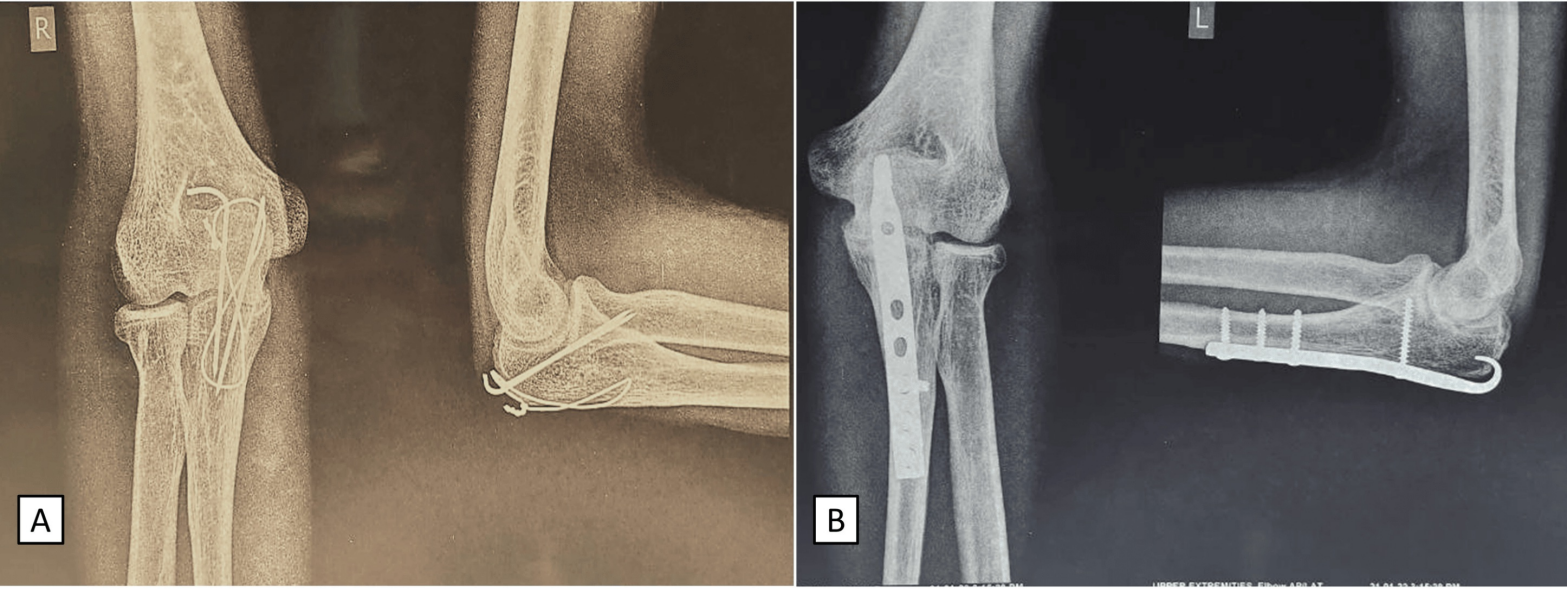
Postoperative radiographs of the left and right elbows taken six months after surgery (A) Radiograph showing the union of the right side, fixed with tension band wiring done for the Mayo type I right olecranon fracture; (B) radiograph showing the union of the left side, fixed with an anatomical plate for the Mayo type II left olecranon fracture.
